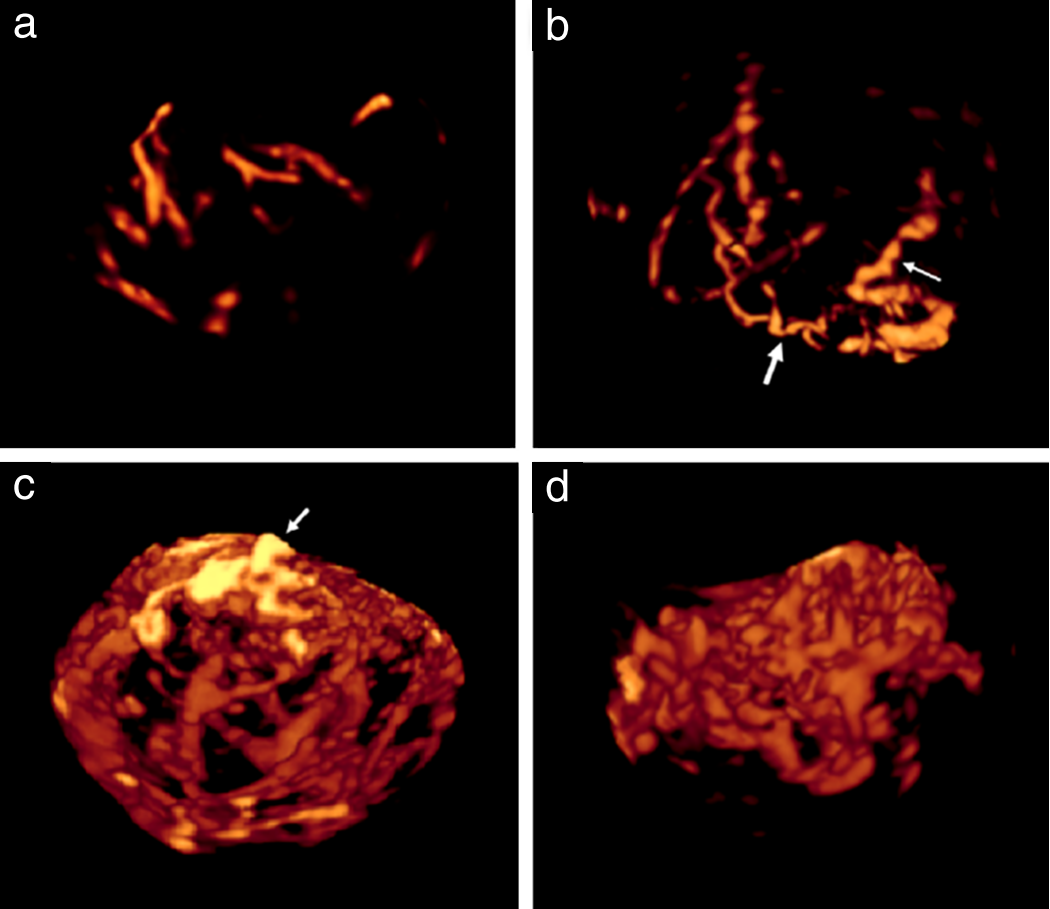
Three‐dimensional power Doppler ultrasound images of vessel tree in whole volume of ovarian tumors, showing: (a) dispersed (as opposed to densely packed), straight (as opposed to tortuous), branching vessels in benign mucinous cystadenoma; (b) dispersed, branching, tortuous vessels (thick arrow) with changes in diameter (thin arrow) in mucinous borderline tumor; (c) densely packed, branching, tortuous vessels with changes in diameter and color splashes (arrow shows splashes) in functional cyst; and (d) densely packed, branching, tortuous vessels with changes in diameter in ovarian clear‐cell cancer. Corresponding rotating images of vessel trees are shown in Videoclips S1–S4.
